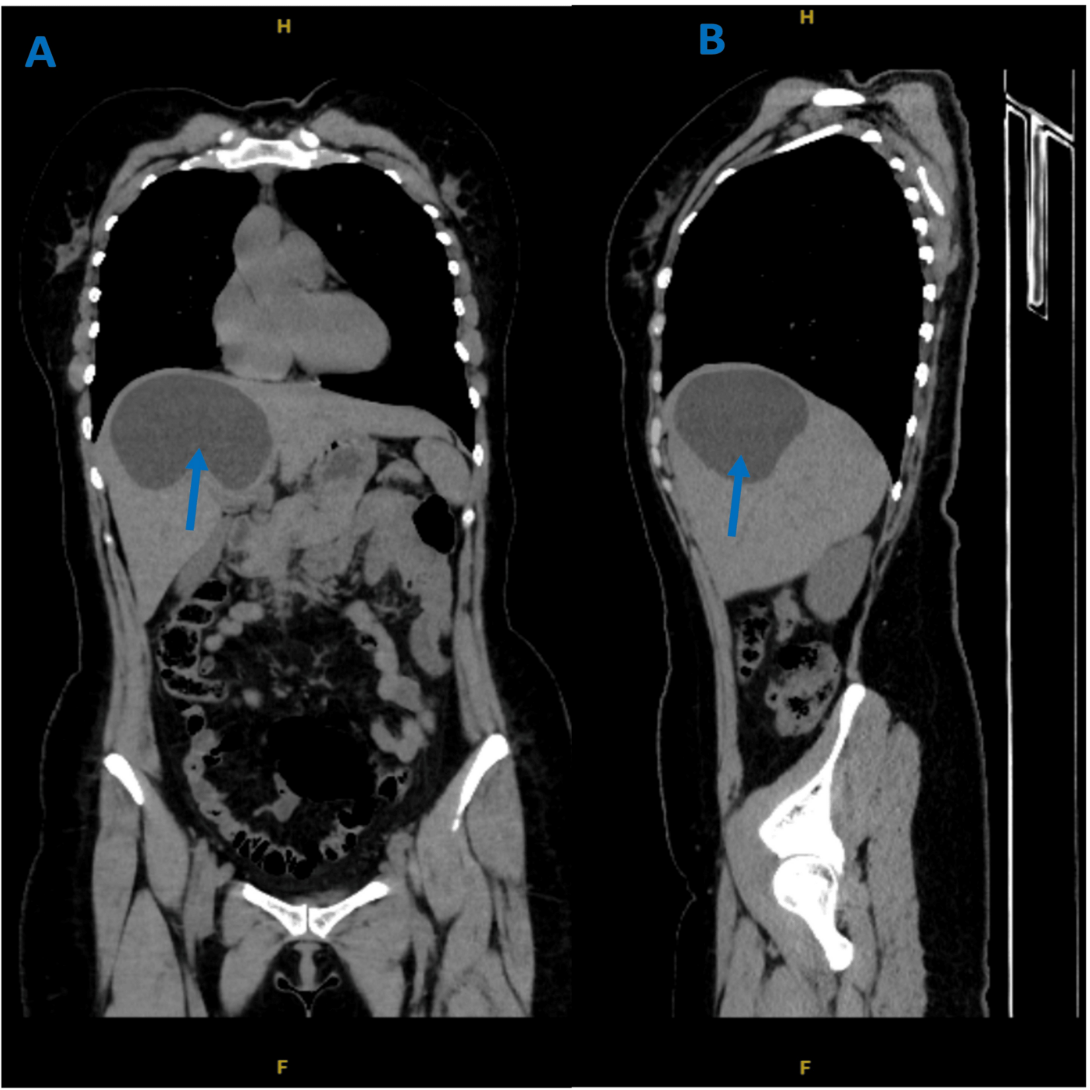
(A) Coronal View of Non-Enhanced Abdominal CT scan and (B) Sagittal View of Non-Enhanced Abdominal CT scan Both scans show hepatomegaly with right hepatic lobe segment VIII and adjoining left hepatic lobe segment IV, a large thick-walled fluid-filled hypodense cystic lesion (blue arrows). CT: Computed Tomography.
